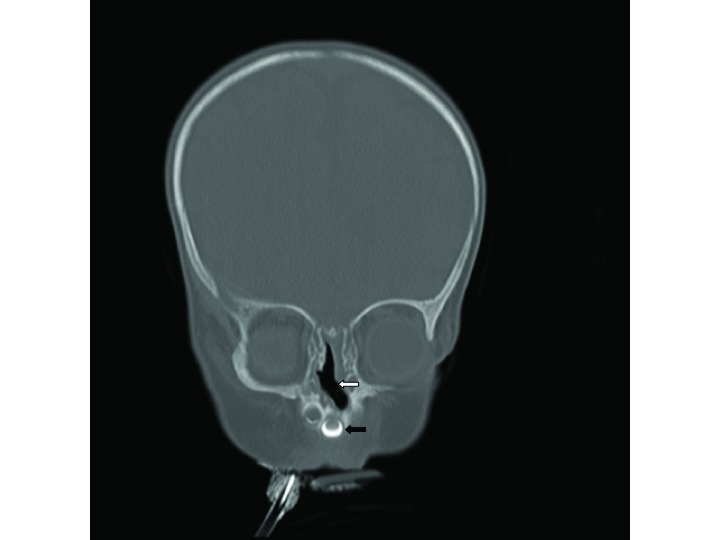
CT image (post-stenting): coronal view of the eroded septum (white arrow) and the central mega-incisor (black arrow).
